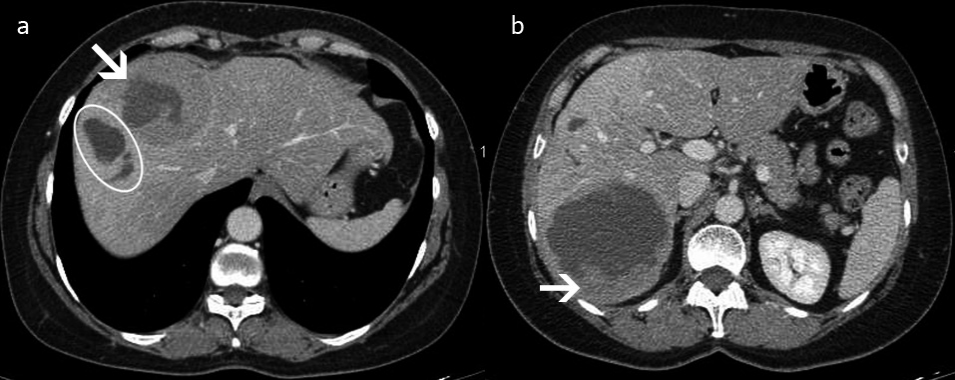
CT of the liver after chemoembolization. Two partially necrotic lesions remained. The first, 8.9 cm in diameter, extended into segments VI and VII, and the second, 4.2 cm in diameter, extended into segments VIII and IV_A_. (a) Arrow indicates the necrosis within the lesion. The circle indicates necrosis of the normal liver parenchyma adjacent to the lesion in segment VIII. (b) The viable tumor at the periphery of the lesion is shown.
